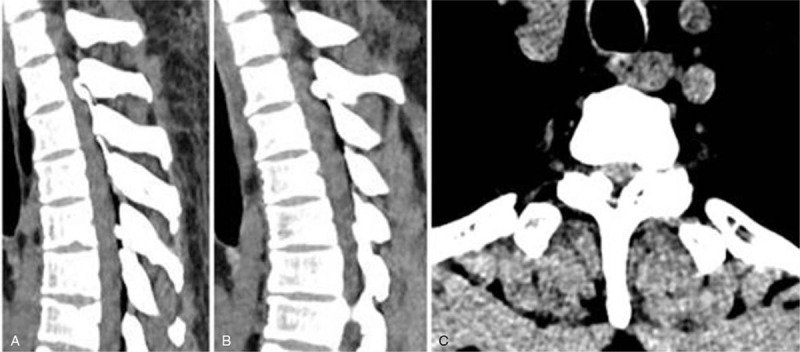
Preoperative CT: T2 and 3 ossification of the ligamentum flavum compression of the spinal cord.
